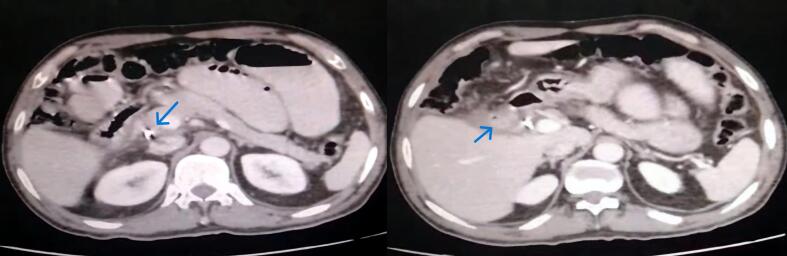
Axial contrast-enhanced CT image demonstrating a cholecysto-duodenal fistula. The image shows direct communication between the gallbladder and the adjacent duodenum (arrow), evidenced by pneumobilia and an indistinct gallbladder wall.
